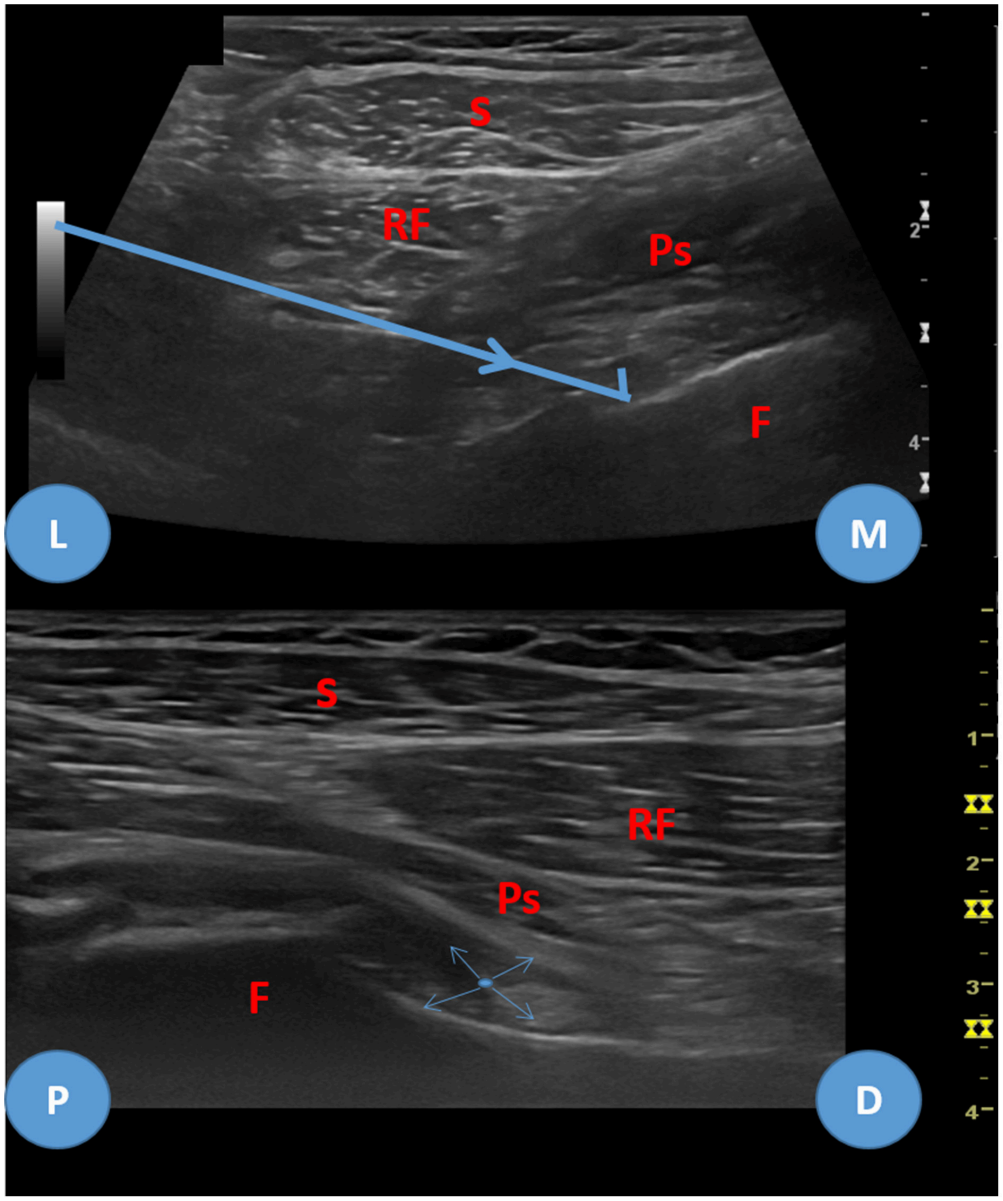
Lateral to medial approach for hip biopsy, ultrasound images. Upper image—transverse-oblique view of the hip joint, L, lateral; M, medial. Bold blue arrow—biopsy needle trajectory. Lower image—longitudinal view, P, proximal; D, distal. Thin blue arrows—needle throw directions. S, sartorius muscle; RF, rectus femoris muscle; Ps, Psoas iliacus muscle and tendon; F, femur.
